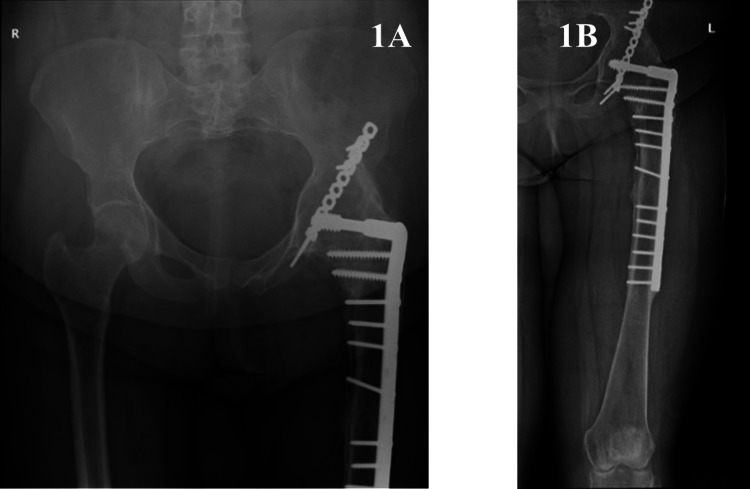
Preoperative plain radiographs of the left hip joint Preoperative plain radiograph of the left knee joint in anterior views showing hip arthrodesis and open reduction and internal fixation of the left proximal femur
